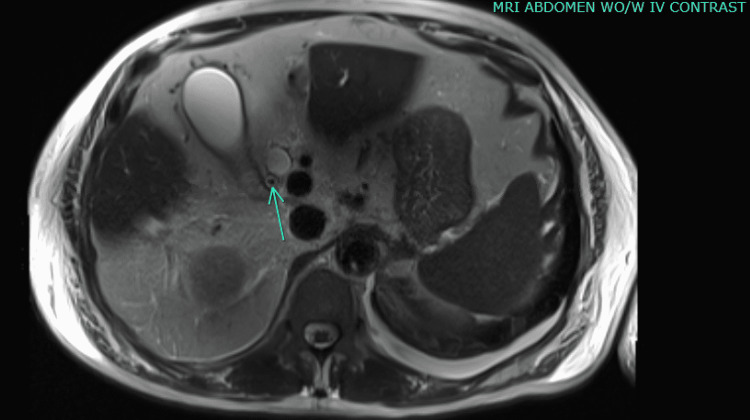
MRI abdomen with and without contrast with magnetic resonance cholangiopancreatography (MRCP) showing a 15 mm dilated distal common bile duct, 10 mm dilated pancreatic duct, and ampulla with a nonspecific 0.6 cm soft tissue density.
